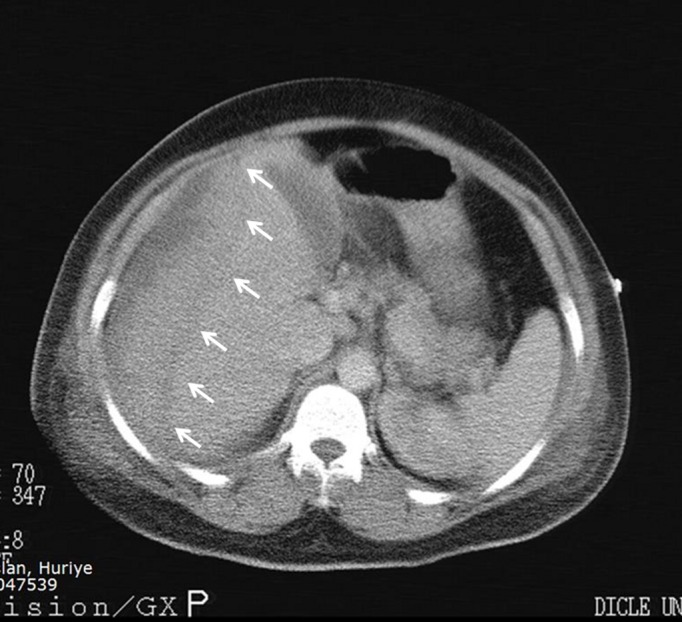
CT shows a subcapsular hematoma in the right hepatic lobe. The capsule is intact.
